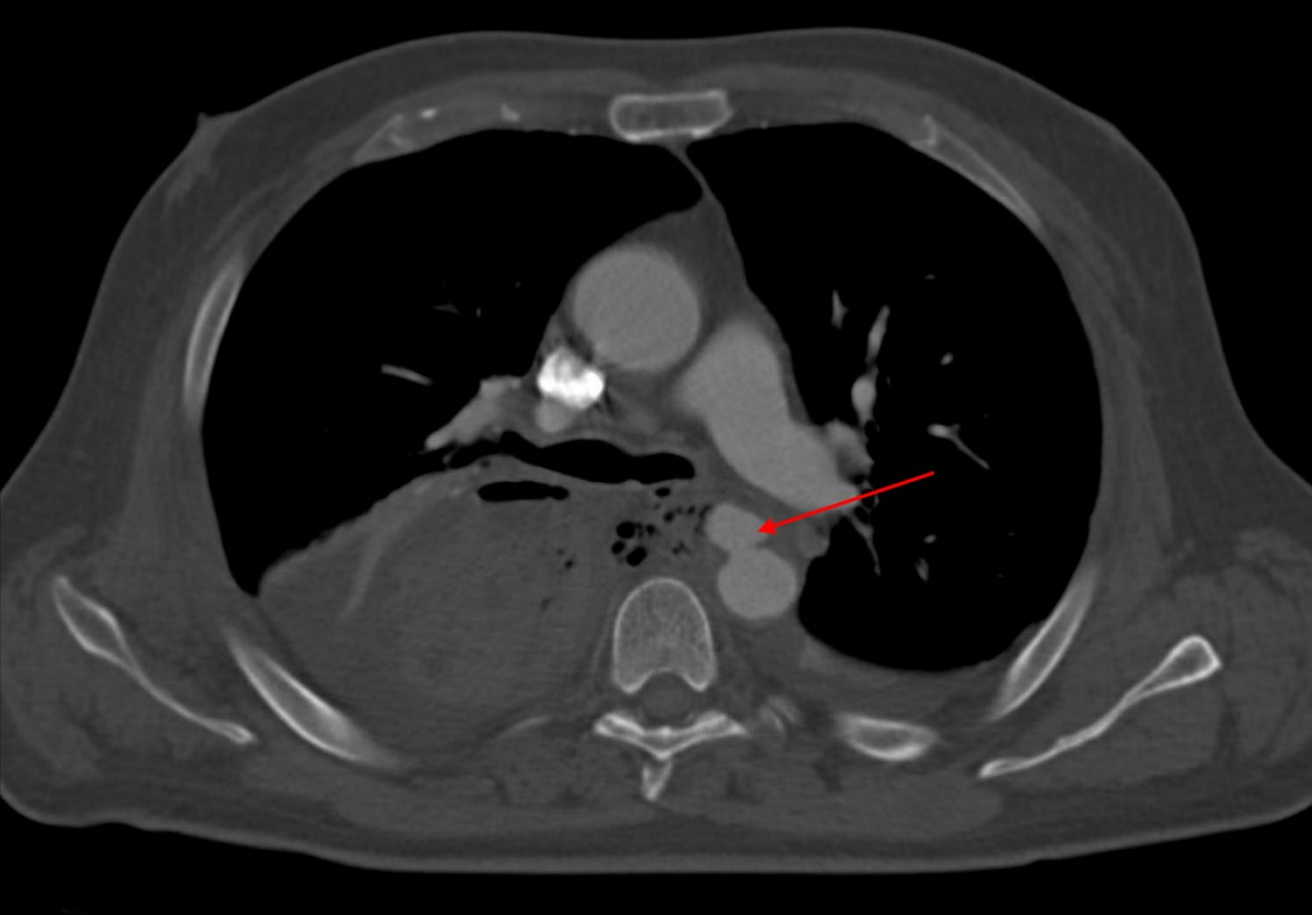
CT angiography showing a pseudoaneurysm in the wall of the thoracic descending aorta adjacent to the gastric conduit (indicated by the red arrow), with adjacent mediastinal air bubbles, indicating mediastinitis
